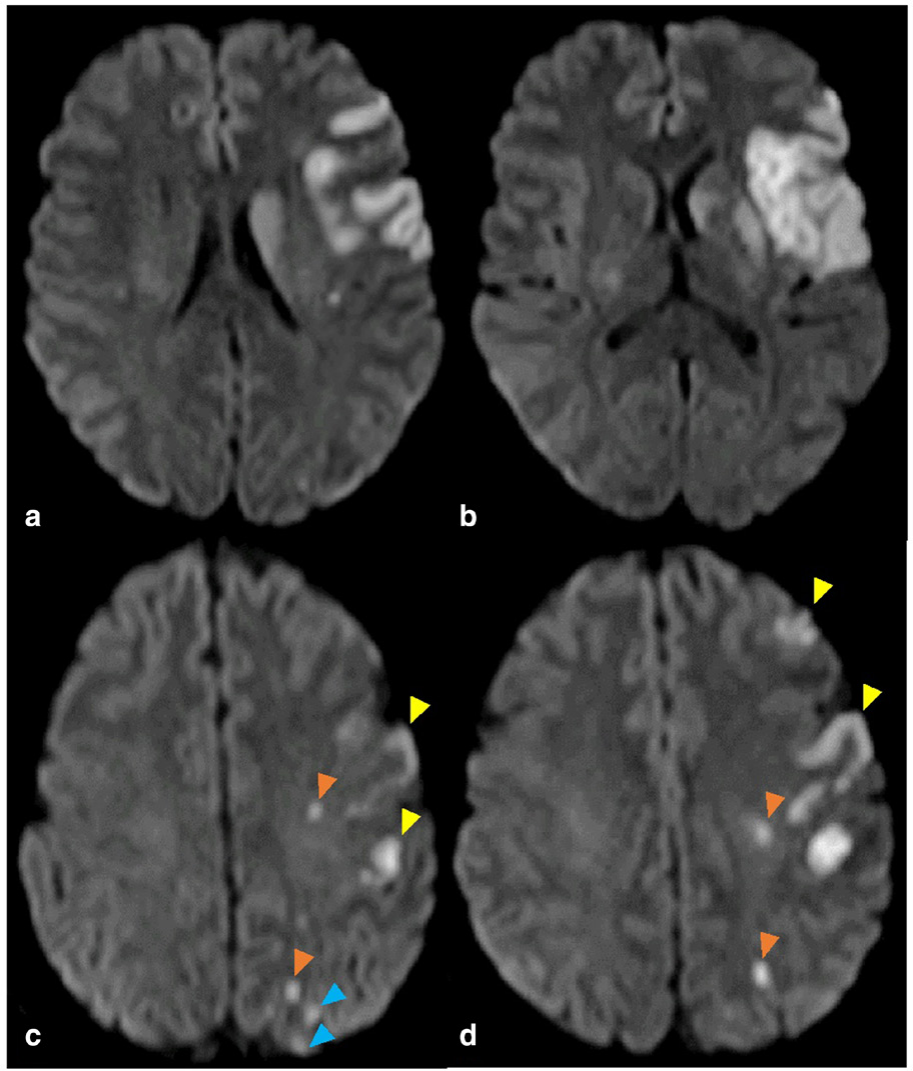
Follow-up MRI diffusion-weighted sequence. Cortical and subcortical infarct in single pial artery territory of left MCA at the ganglionic level (a, b). Embolic-type left MCA territory cortical infarcts at supraganglonic level (yellow arrowheads). Punctate infarcts in internal subcortical (orange arrowheads) and external cortical (blue arrowheads) watershed zones between left ACA/MCA vascular territories (c, d). ACA, anterior cerebral artery; MCA, middle cerebral artery.
